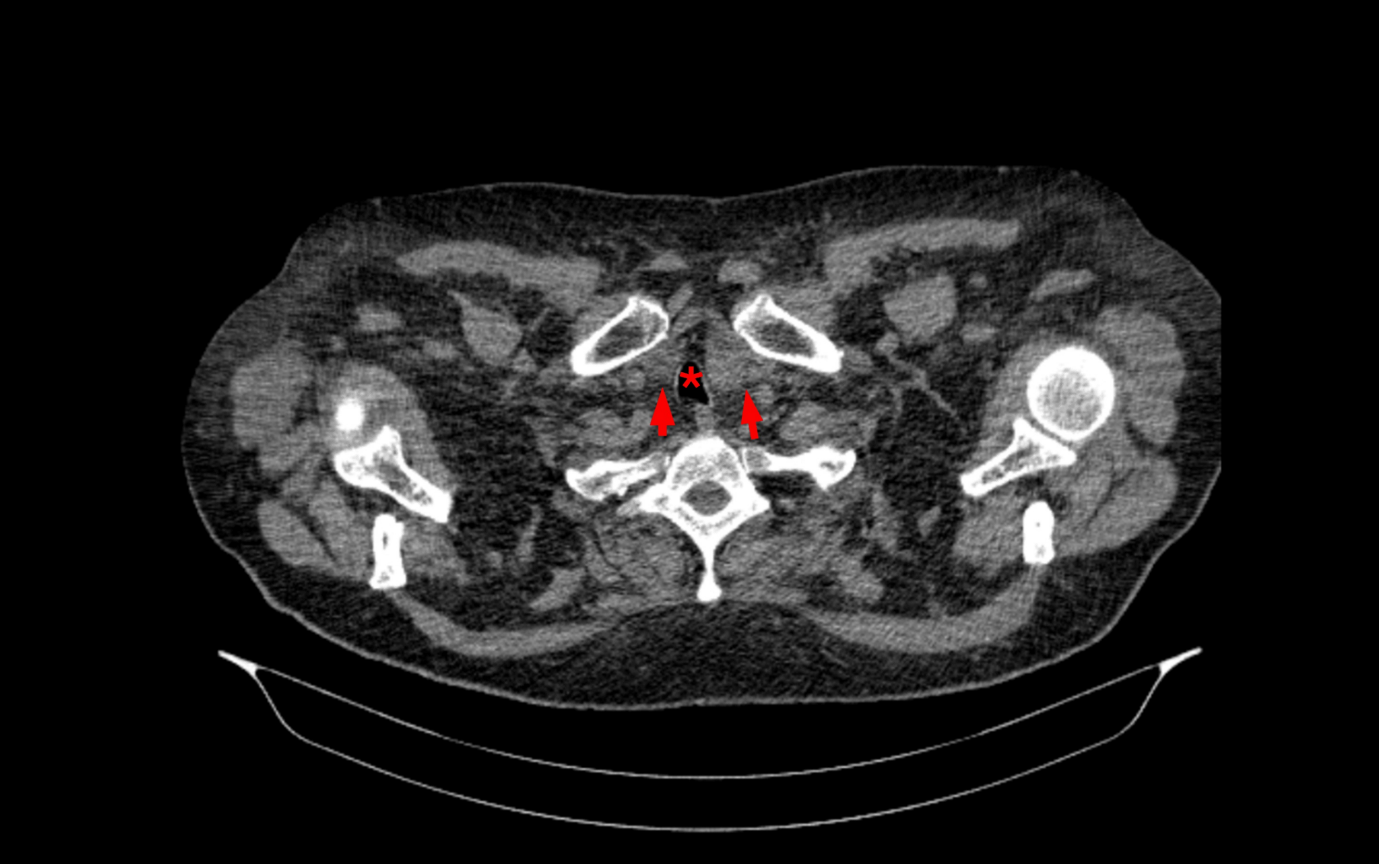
Cervical-thoracic CT scan reveals thyroid enlargement, denoting retrosternal goiter (red arrows) and tracheal compression (red "asterisk" - trachea).
